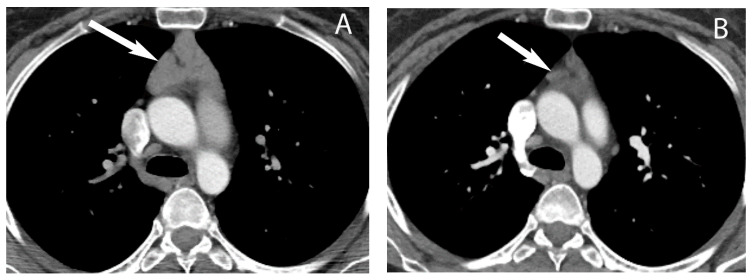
Thymic hyperplasia. CT (A) shows diffuse enlargement of the thymus maintaining its triangular or bilobed shape (arrow). Follow-up CT (B) 12 months later shows fatty involution of the thymus (arrow).
